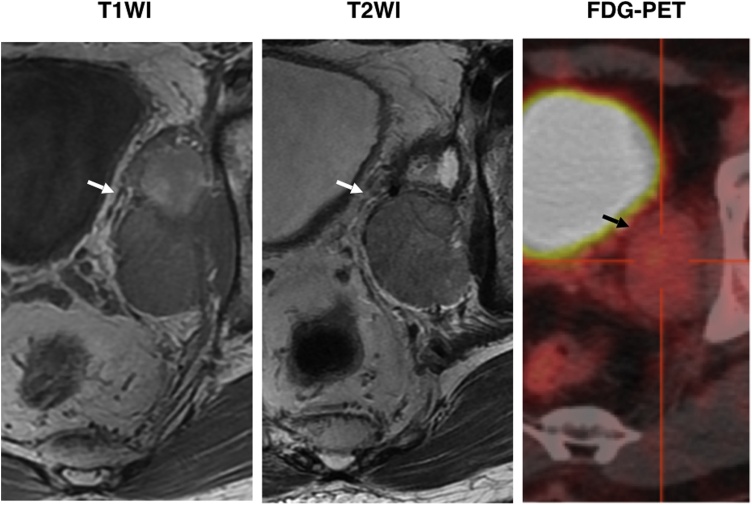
Preoperative MRI and FDG-PET findings of Case 2. Preoperative MRI after intraoperative biopsy revealed a well-demarcated, T1 and T2 low intensity tumor. In FDG-PET, an accumulation of SUV max 2.3 (im259) corresponding to a maximum length 40 mm mass was identified.
